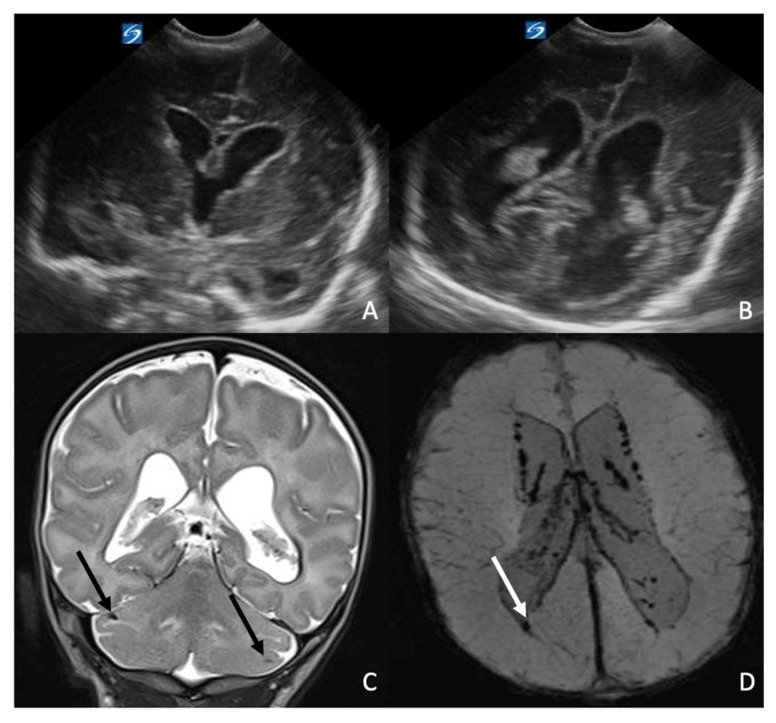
The US performed at 15 days of life (A,B) and an MRI performed at 20 days of life in a girl diagnosed with *Listeria monocytogenes* infection. The coronal US images (A,B) show mild asymmetry of the lateral ventricles with slight left predominance, associated with third-ventricle dilatation and diffusely hyperechoic ependyma, consistent with ventriculitis and prior subependymal haemorrhage, as well as increased echogenicity of the periventricular white matter. The subsequent brain MR, particularly coronal T2WI (C) and axial SWI (D), confirms subependymal haemorrhage, seen as irregular hypointensity along the ventricular walls, and shows associated mild intraventricular haemorrhage (white arrow in (D)) and cerebellar haemorrhagic foci (black arrows in (C)).
